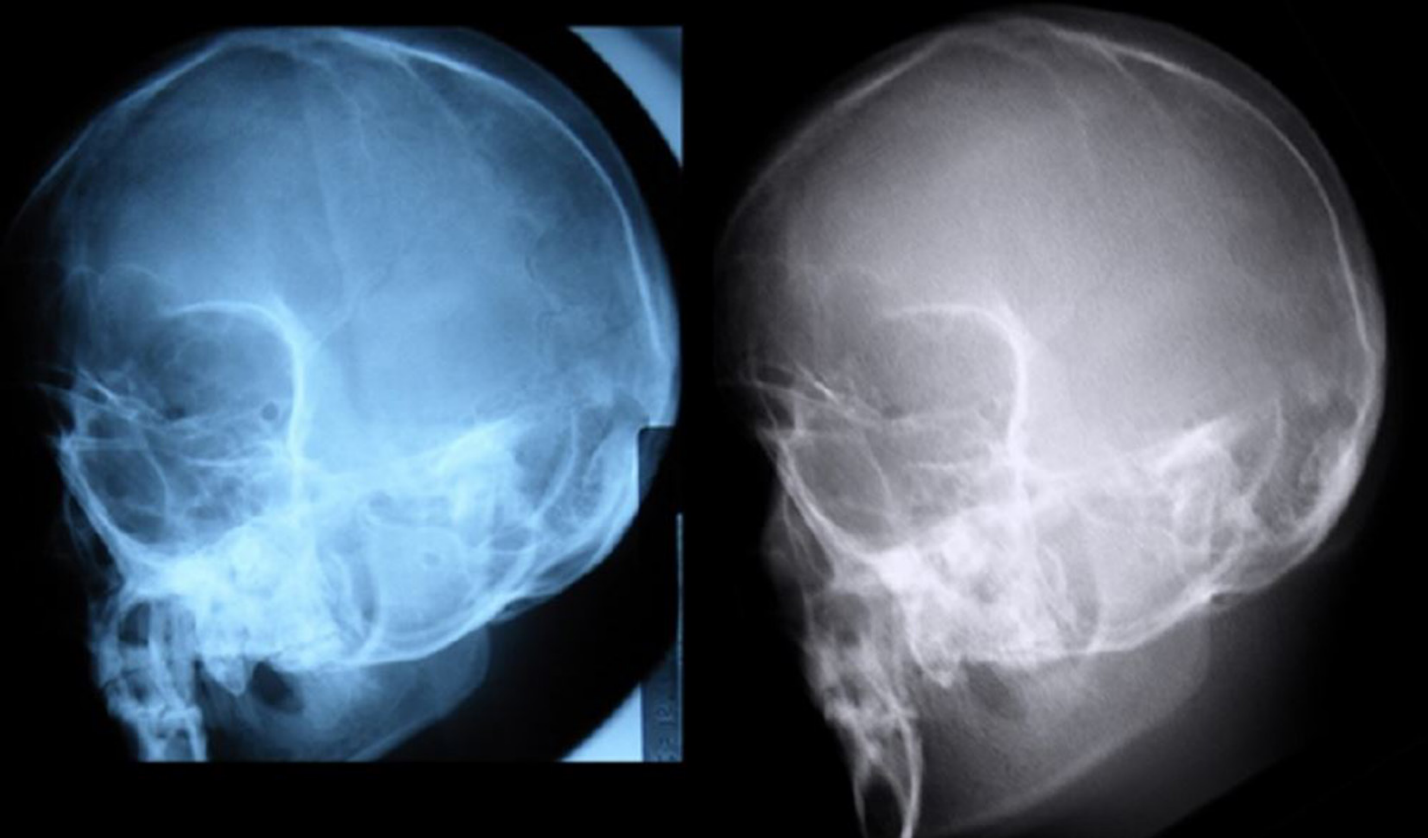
(a) real and (b) virtual radiograph Optic Foramina projections compared.
